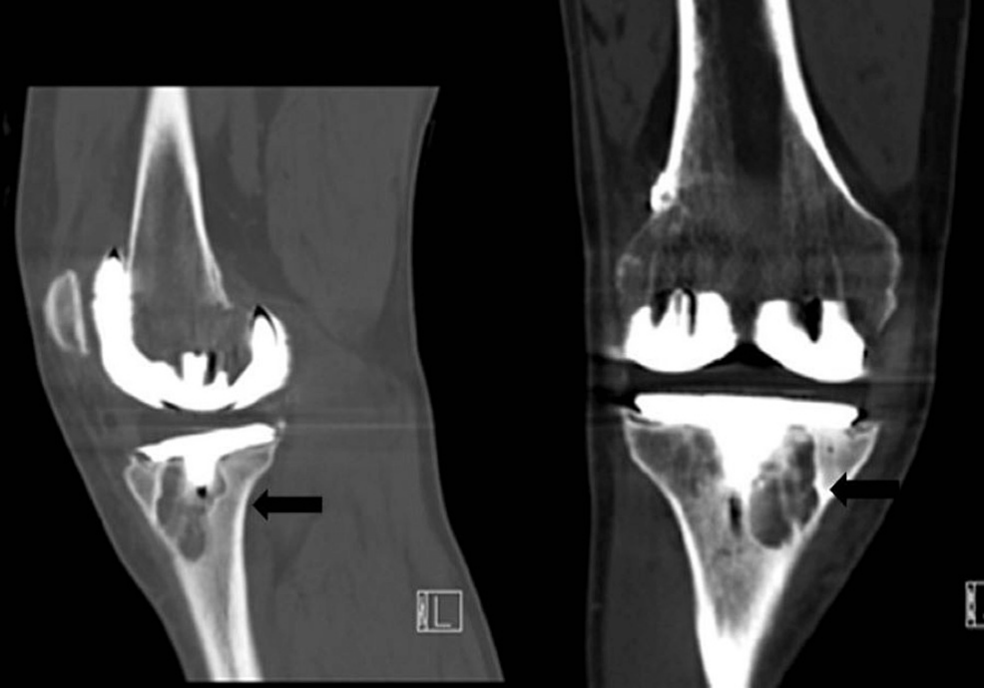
CT scan images. Depicting osteolysis around the tibial component in nine coronal and sagittal views indicated by arrows around the tibial stem. CT, computed tomography
